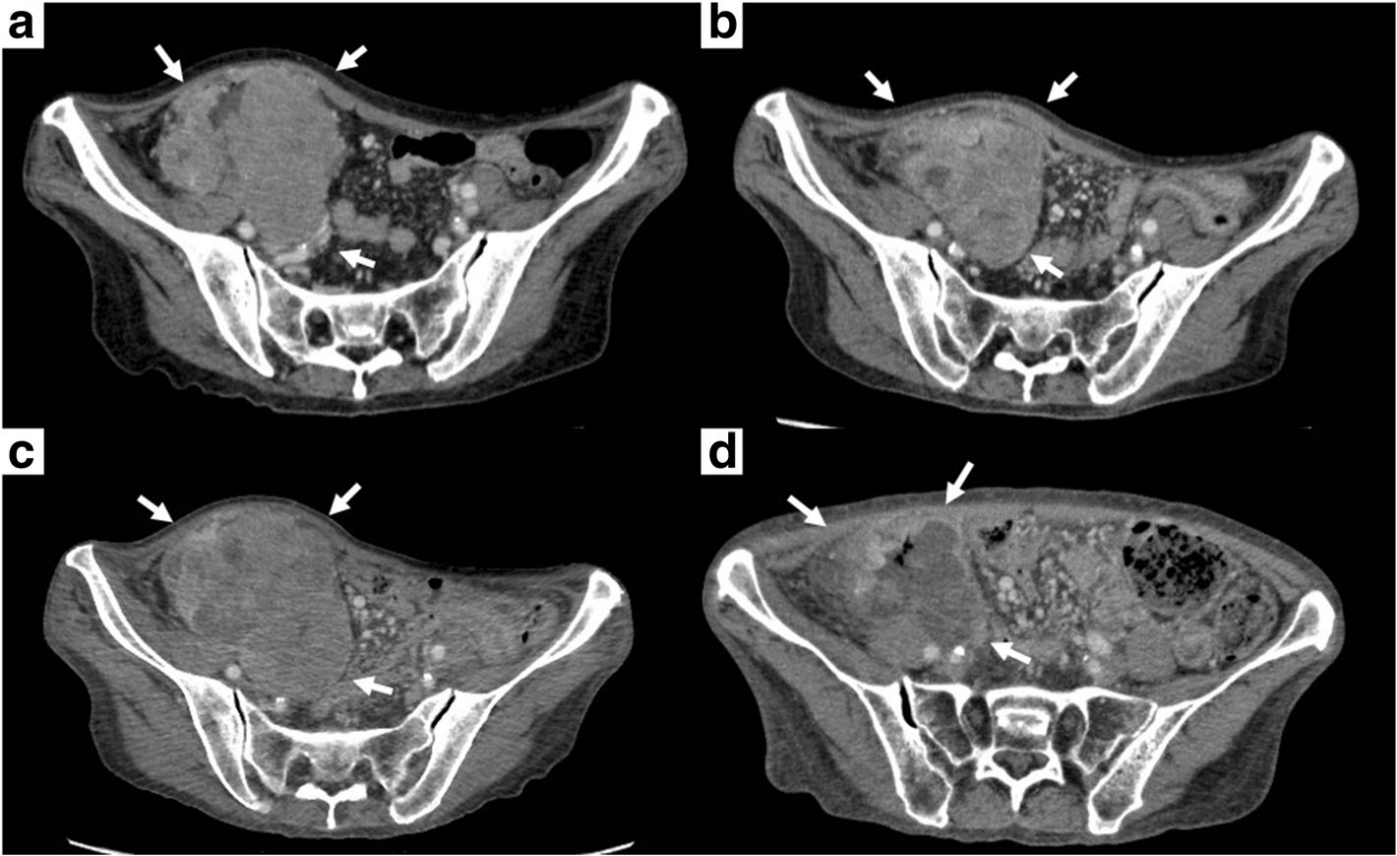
Computed tomography images. a Before treatment, 8.6-cm-diameter tumor infiltrating the abdominal wall, small intestine, and retroperitoneum. b After four cycles of chemotherapy, the tumor slightly shrank to 6.9 cm in diameter. c After eight cycles of chemotherapy, the tumor enlarged to 10 cm in diameter. d After radiotherapy, the tumor reduced to 6.6 cm in diameter with intratumor liquefaction degeneration
